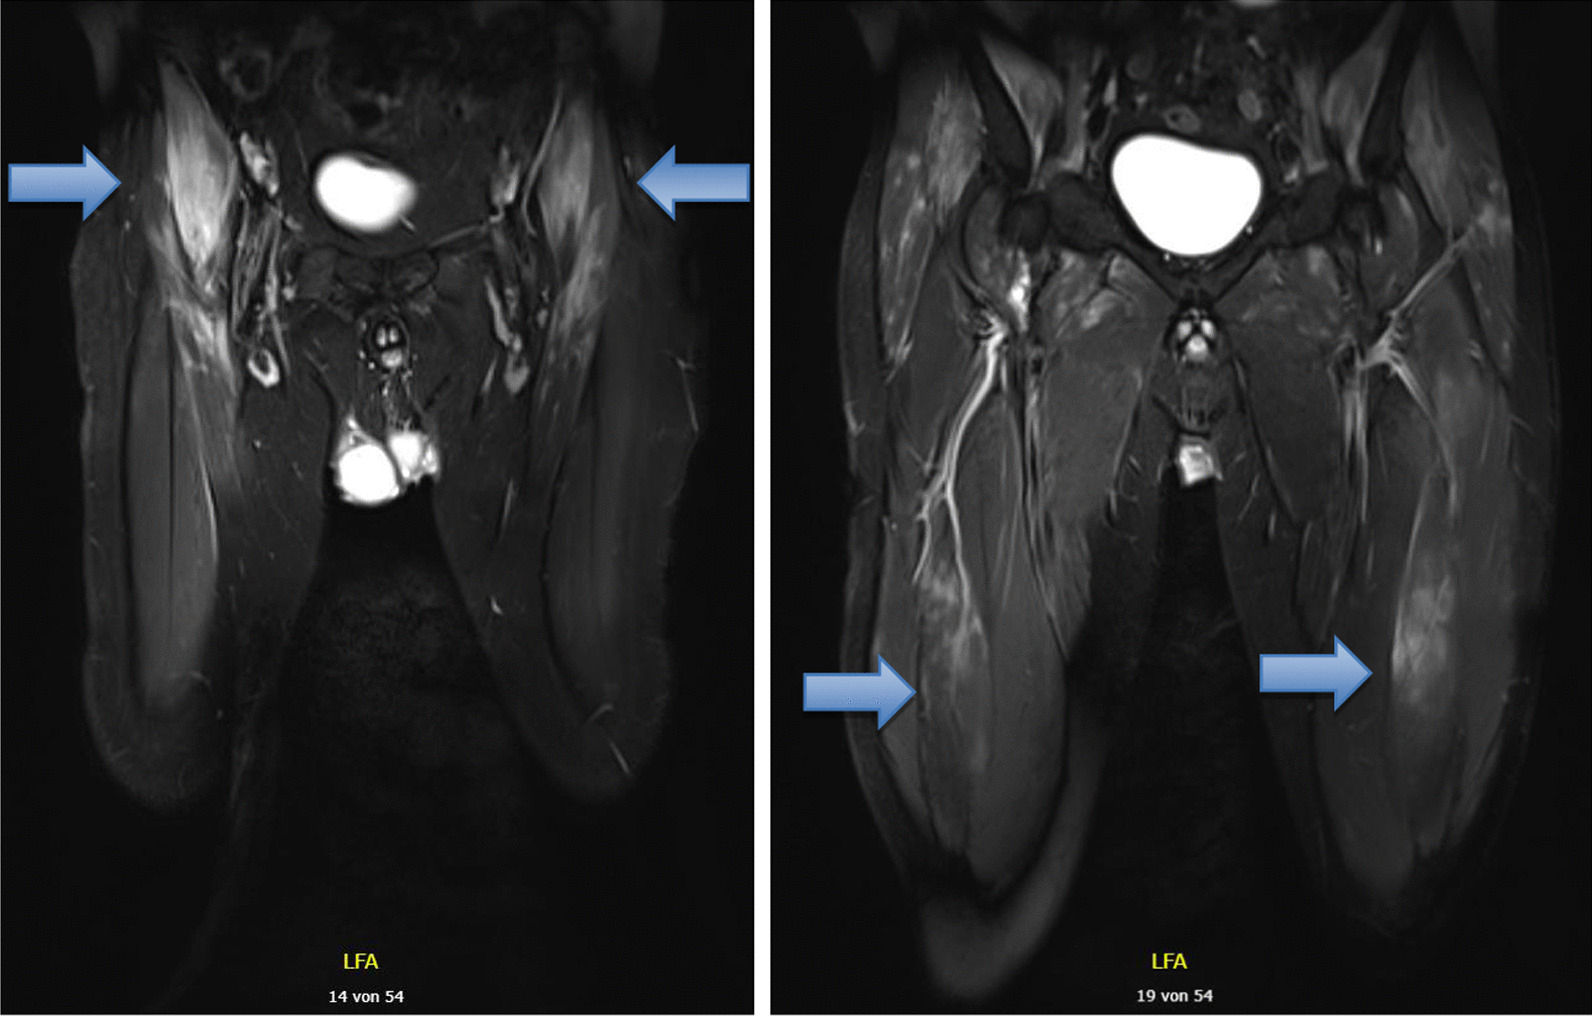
3-Tesla, gadolinium contrast enhanced MRI, T2 sequence at week 3 revealed bilateral signal enhancement in tensor fasciae latae, sartorius muscles, gluteus minimus, iliopsoas muscles (blue arrows) and other thigh muscles, with maximal involvement of the right iliopsoas muscle. The image also shows increased number and borderline size of the (reactive) inguinal lymph nodes bilaterally
